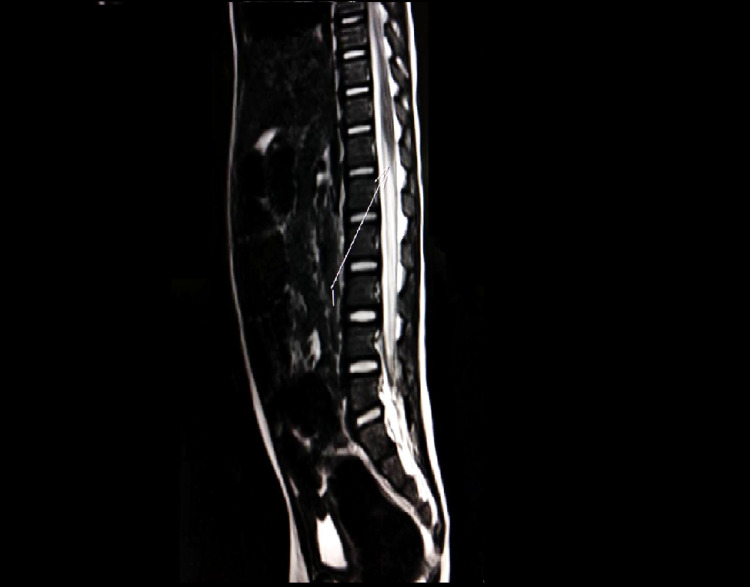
Saggital section MRI spine showing thickened conus medullaris and cauda equina nerve root with intense post-contrast enhancement MRI: magnetic resonance imaging
